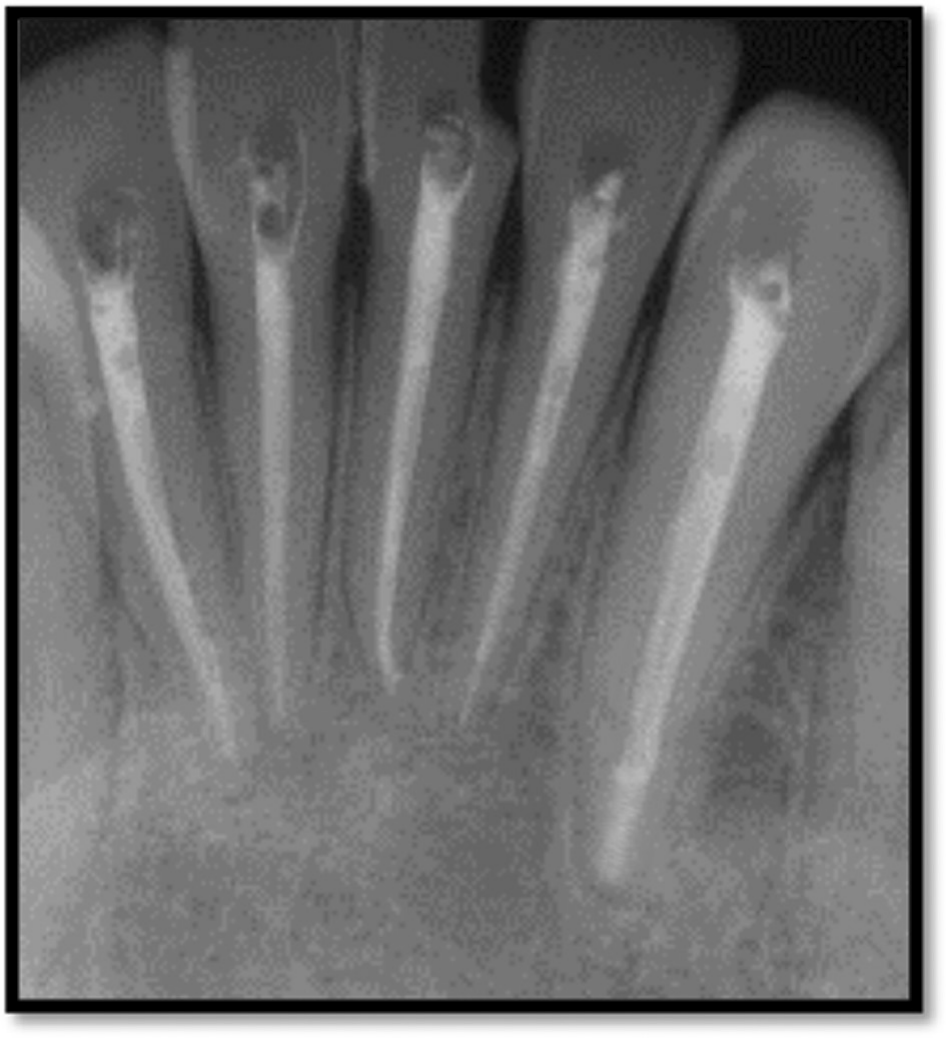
Fifteen‐month follow‐up radiograph post‐obturation.
